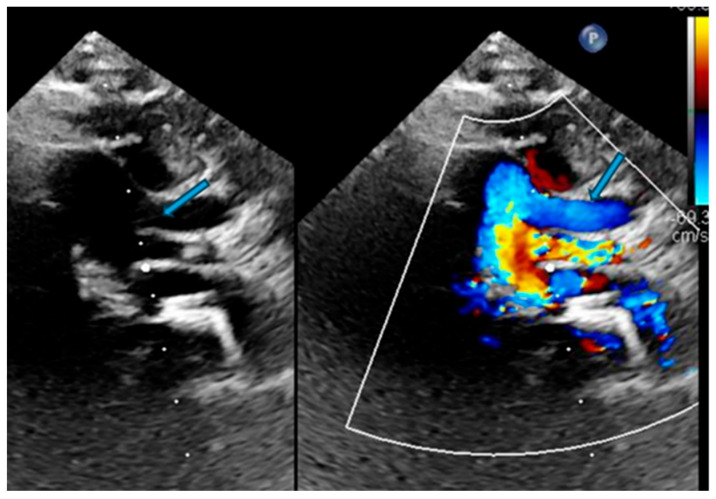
Echocardiogram image showing tortuous aortic arch branches (blue arrow) in a patient.
